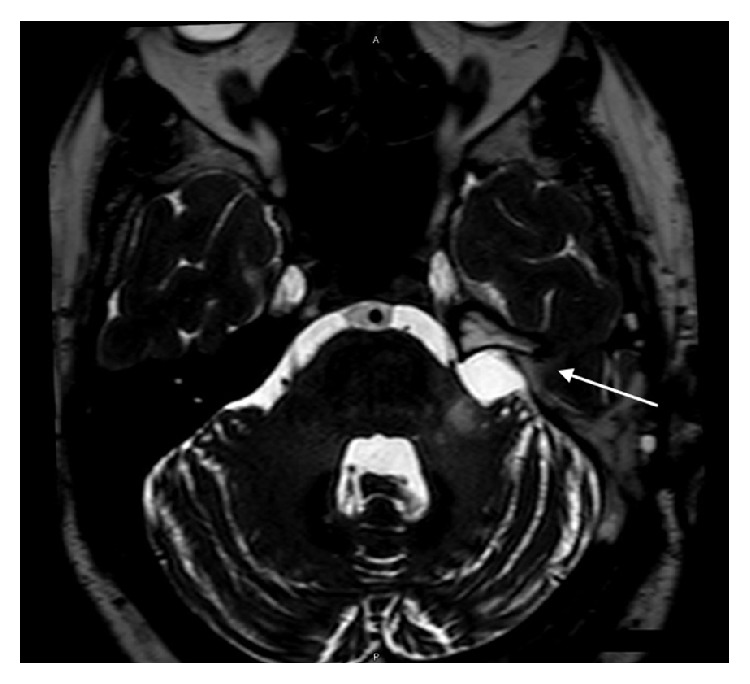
Axial MR image (T2 weighted, 3D DRIVE CLEAR) showing the postoperative changes.
